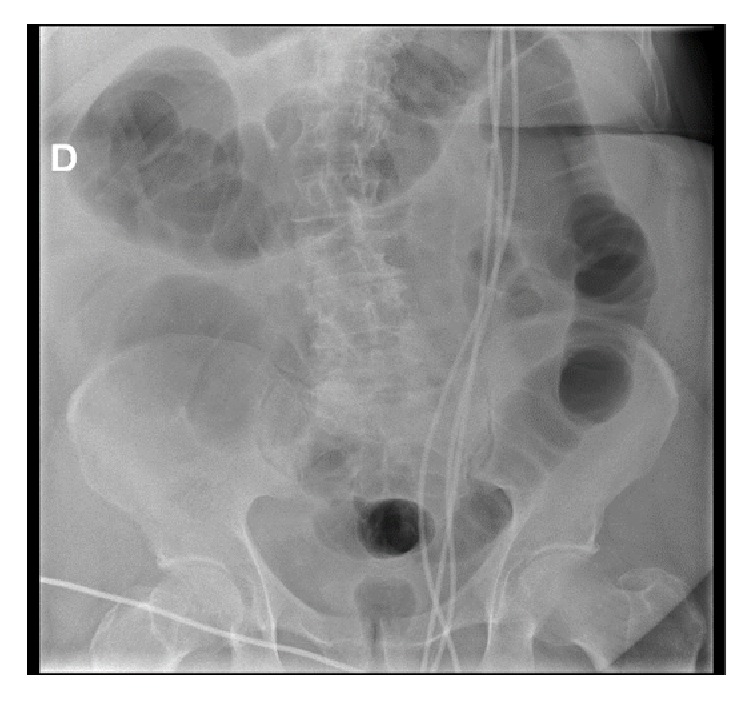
Abdomen X-ray on admission showing diffuse intestinal distension.
